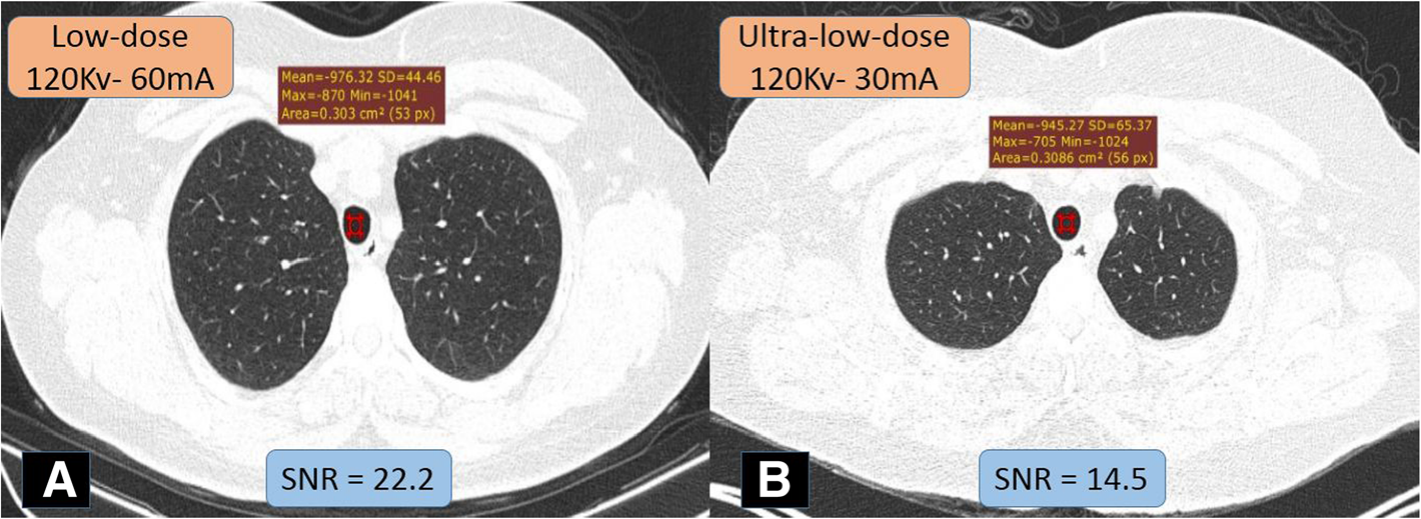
Image quality at highest and lowest SNR; measured at axial chest CT cuts/lung window (− 700:1500) within the tracheal lumen immediately above the aortic arch at standardized 0.3 cm^2^ ROI (SNR = mean attenuation/SD). a Low-dose CT with higher SNR in a 77-kg patient and b ultra-low-dose CT with lower SNR in a 85-kg patient; there is no significant blurring at lung image; however, mild blurring is noted at the shadow of the thoracic cage muscles
